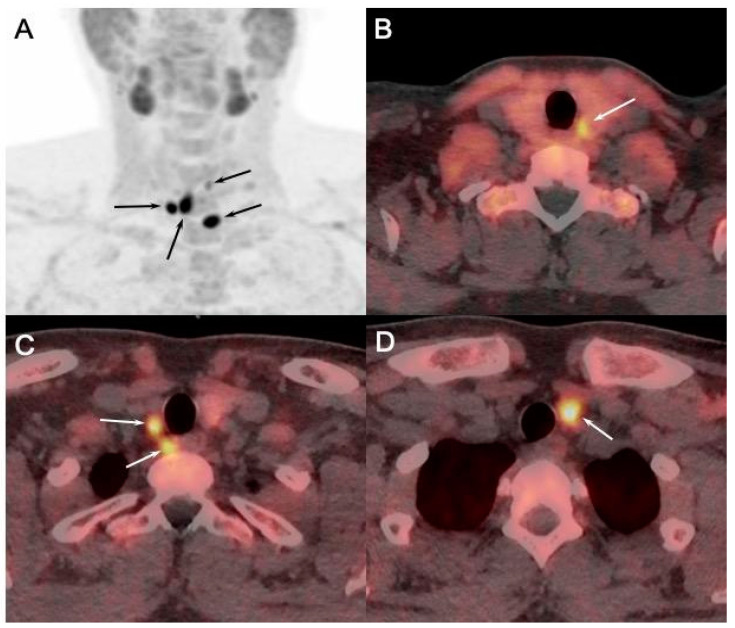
[^18^F]FCH PET/CT ((A): anterior MIP; (B–D): axial fusion images) performed in 23-y-old MEN1 patient with asymptomatic primary hyperparathyroidism (PTH: 389 ng/L; calcemia: 2.71 mmol/L) showing pathologic [^18^F]FCH uptake in both superior and inferior parathyroids (arrows). Pathology after surgical excision confirmed presence of parathyroid adenomas.
